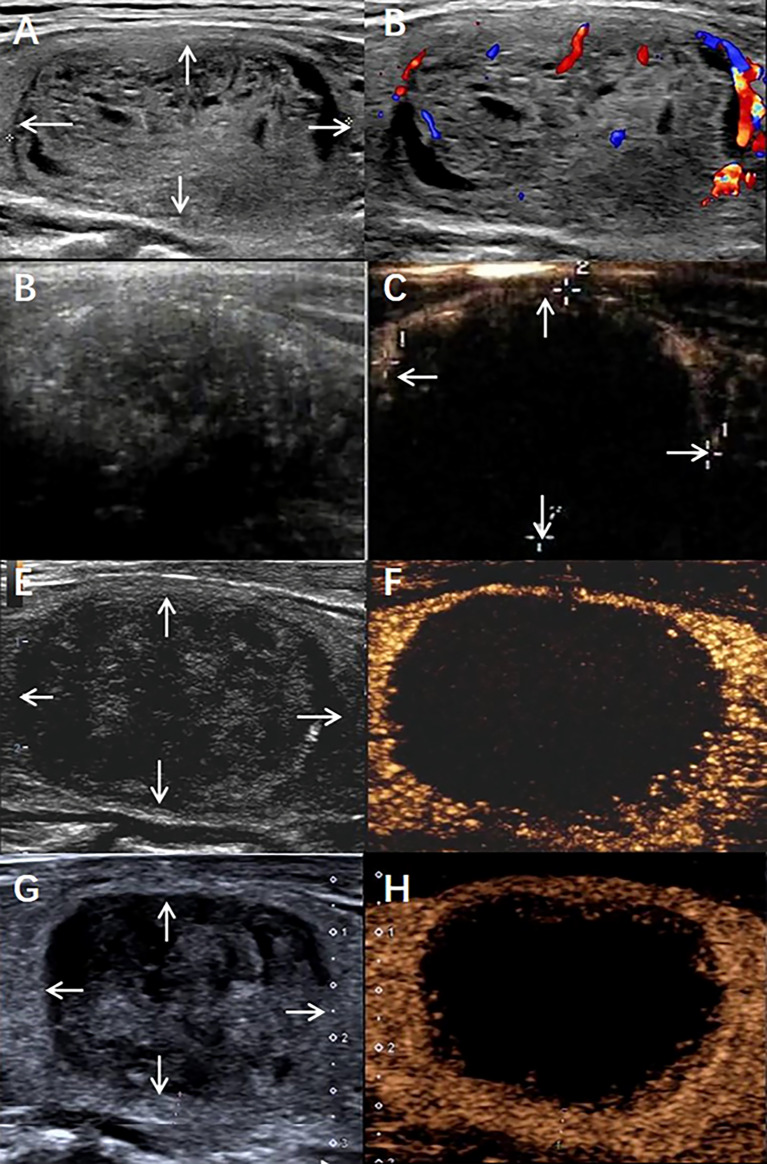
A benign thyroid nodule in a 58-year-old male treated with radiofrequency ablation (RFA). (A) The nodule was in the patient’s right lobe. Arrows indicate the boundaries of the nodule The longest diameter was 4.2 cm, and the volume was 21.4 mL. (B) Colour ultrasound imaging of nodule. (C) Conventional ultrasound imaging of nodule Immediate after radiofrequency ablation. (D) Contrast-enhanced ultrasound imaging of nodule Immediate after radiofrequency ablation. Arrows indicate the boundaries of the treated area. The longest diameter treated areas was 4.1cm and the volume was 17.7mL, IRAR is 83.7%. (E) Conventional ultrasound imaging of nodule 6 months after radiofrequency ablation. The longest diameter was 3.2cm, the total volume of nodule was 12.7mL, VRR is 40.6%. (F) Contrast-enhanced ultrasound imaging of nodule 6 months after radiofrequency ablation. (G) Conventional ultrasound imaging of nodule 12 months after radiofrequency ablation. The longest diameter was 2.5cm, the total volume of nodule was7.67mL., VRR is 65.2%. (H) Contrast-enhanced ultrasound imaging of nodule 12 months after radiofrequency ablation.
